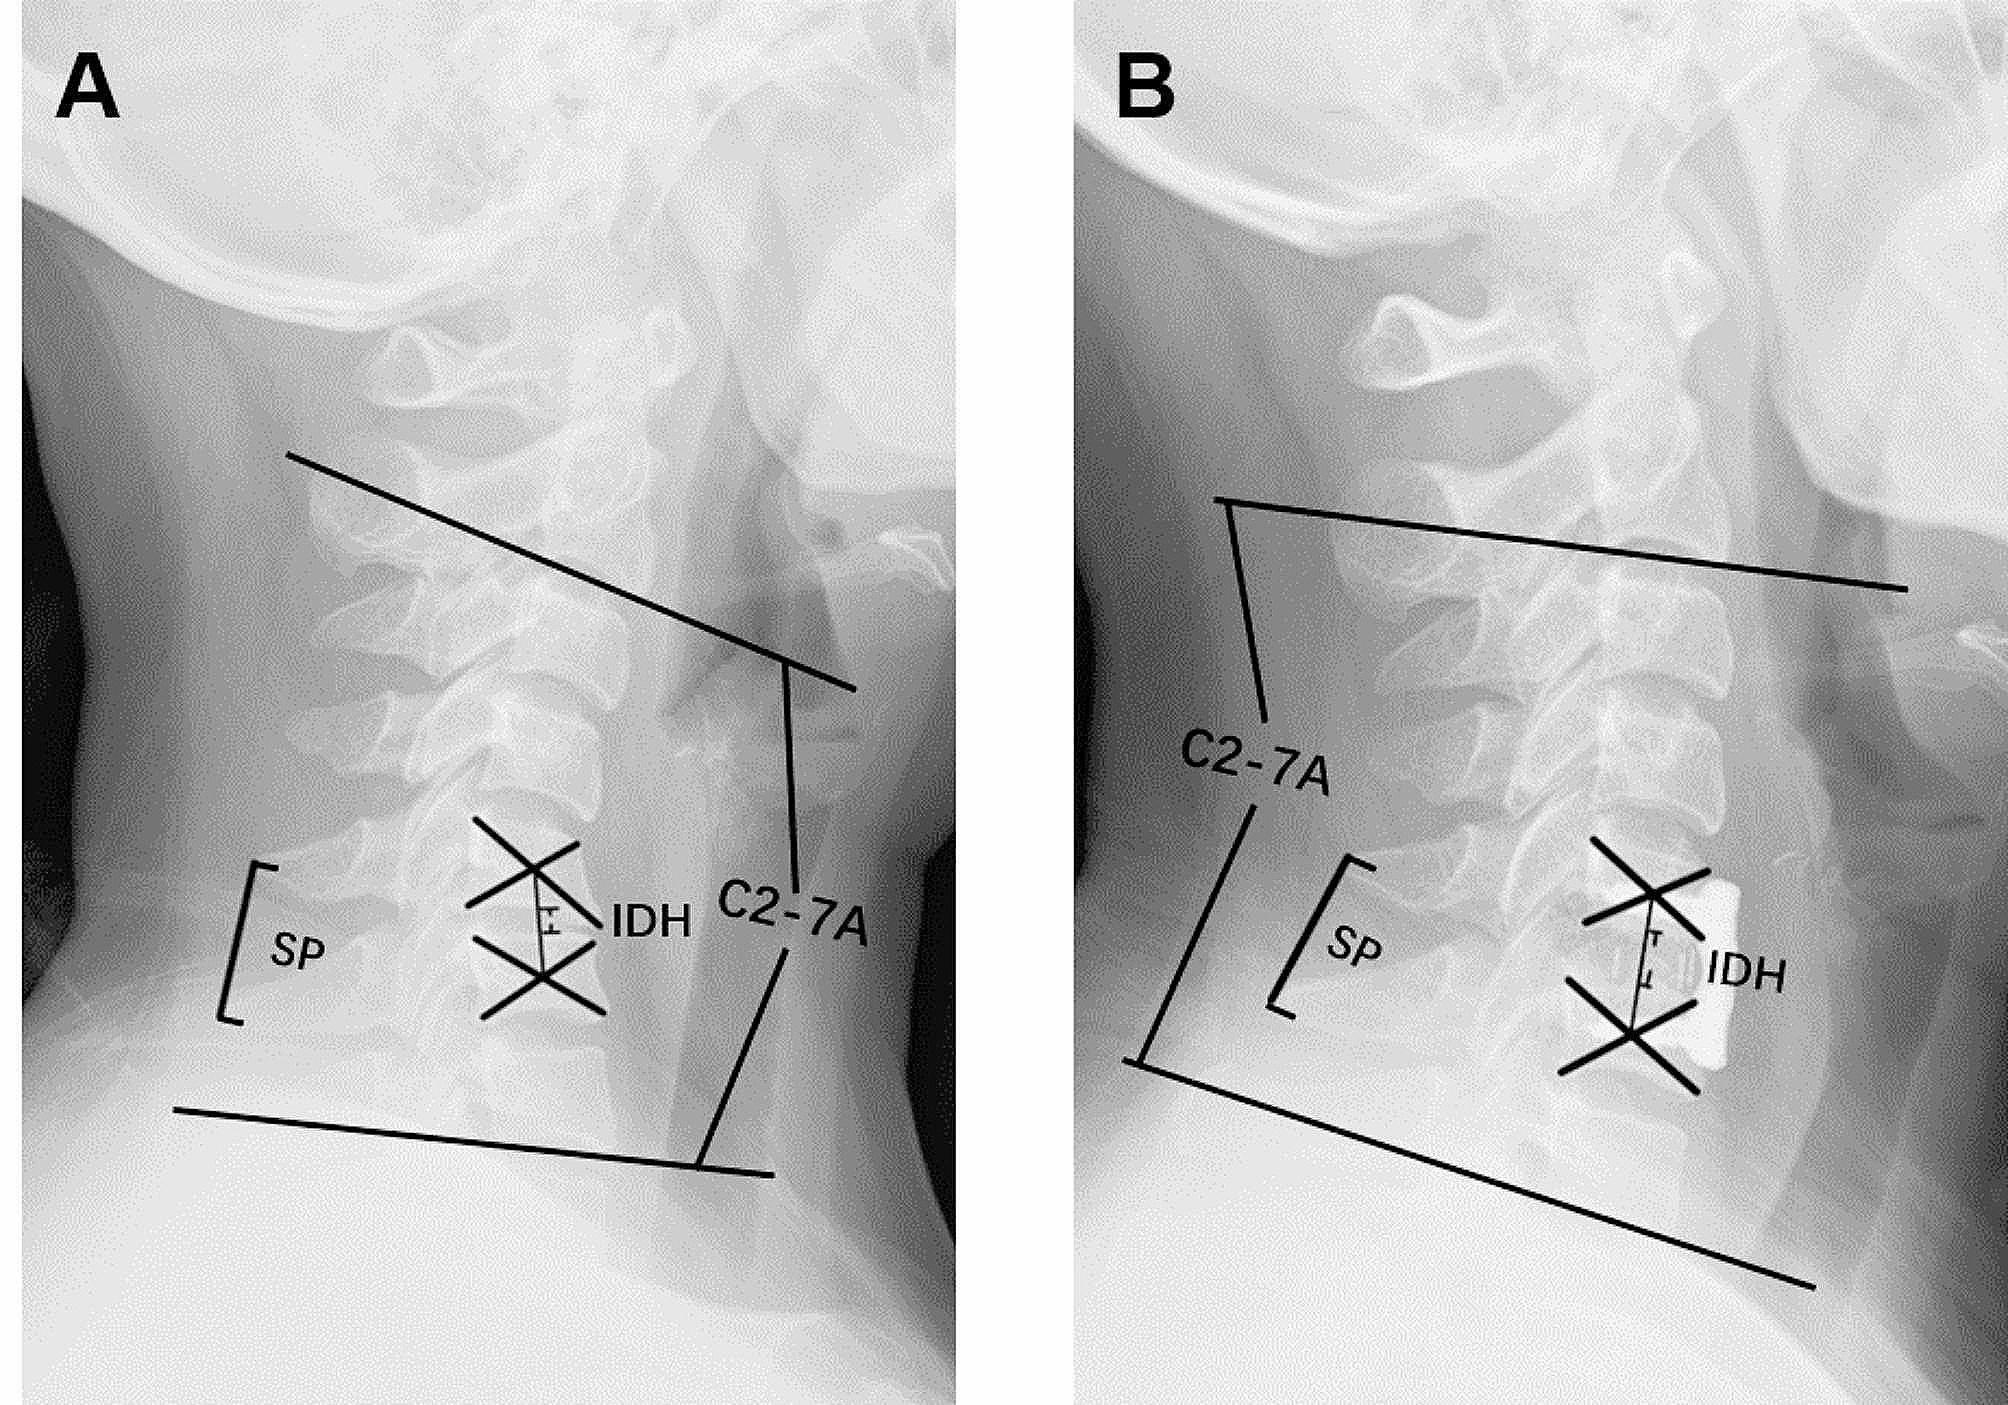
The definition of radiographic parameters on lateral radiographs, preoperatively (A) and postoperatively (B). C2-7 A was defined as the angle between the sacral endplates of C2 and C7; IDH was defined as IDH = the distance between the center of the upper and lower vertebrae - the distance between the center of the upper vertebral body and its lower endplate - the distance between the center of the lower vertebral body and its upper endplate. SP was the distance between the most posterior and caudad point of one spinous process to the same point on the adjacent spinous process
